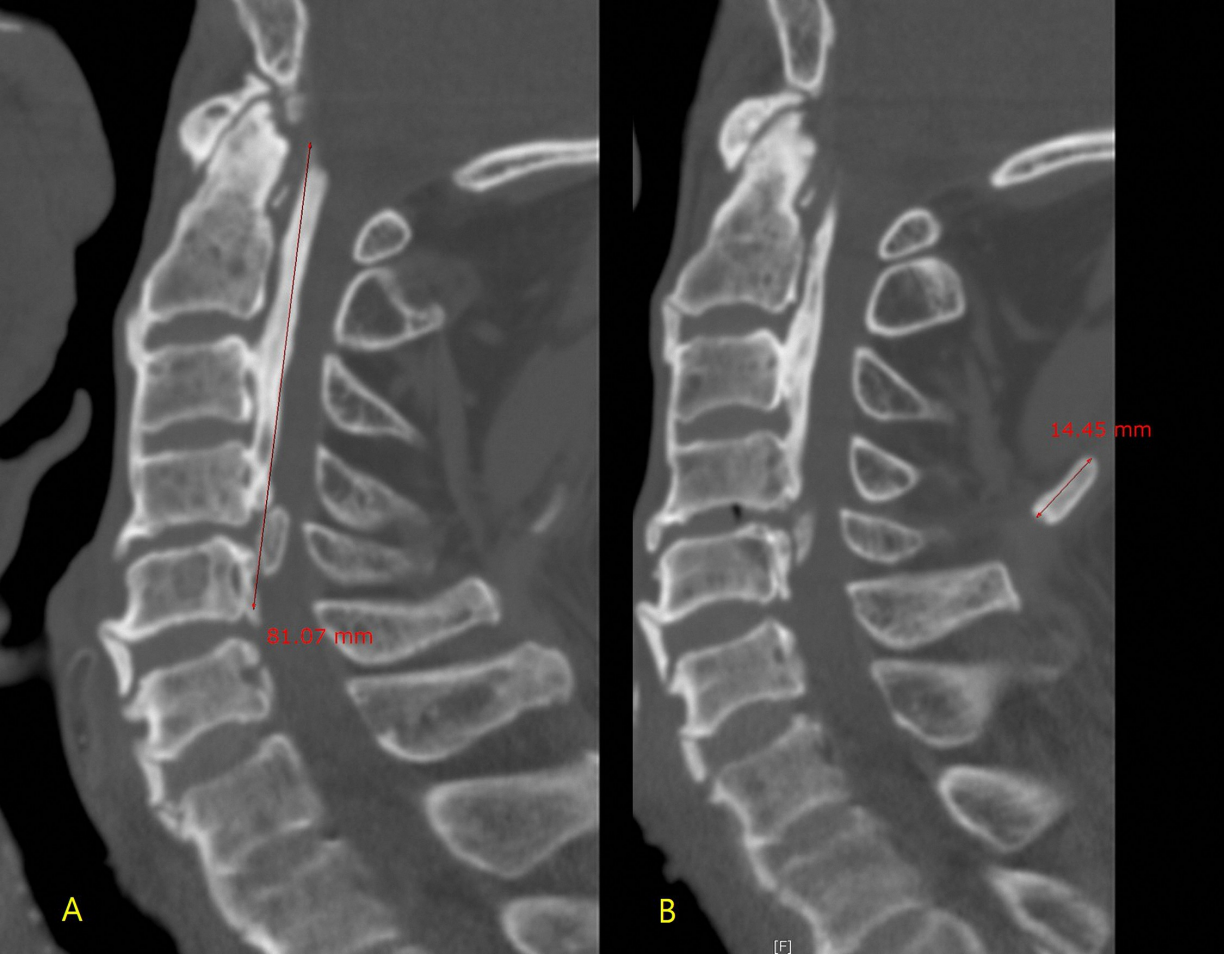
66-year-old man with neck pain and mobility disturbance after trauma. (A) The sagittal reconstructed CT image revealed ossification of the posterior longitudinal ligament extending from C1 to C5 and measuring 8.1cm along its entire length. (B) Another CT image showed ossification of the nuchal ligament extending from C4 to C5 and measuring 1.4cm along its entire length.
